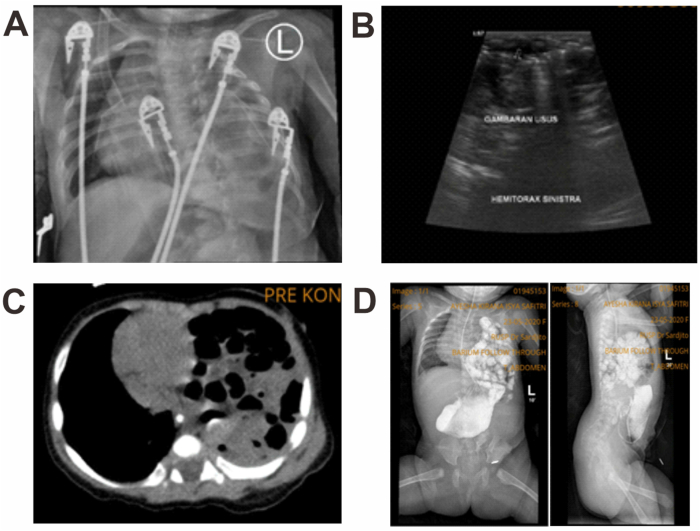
A. Chest X-ray at the age of 4 months showed a lucent lesion with septation in the left thoracic cavity; B) Thoracic ultrasound showed the left thoracic cavity filled with intestines; C) CT scan of the thorax showed elevated small intestine and colon which pushed the mediastinum to the contralateral (right) side; and D) X-ray of small bowel follow-through (AP and lateral position) showed the intestines in the left thoracic cavity.
